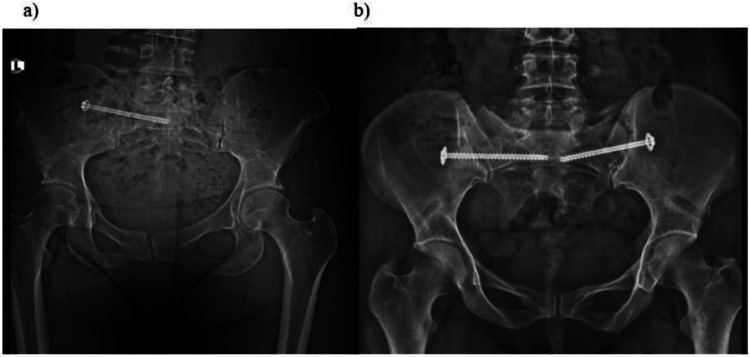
Anteroposterior pelvic radiographs showing sacroiliac screw fixation configurations. (a) Unilateral sacroiliac screw fixation. (b) Bilateral sacroiliac screw fixation.
